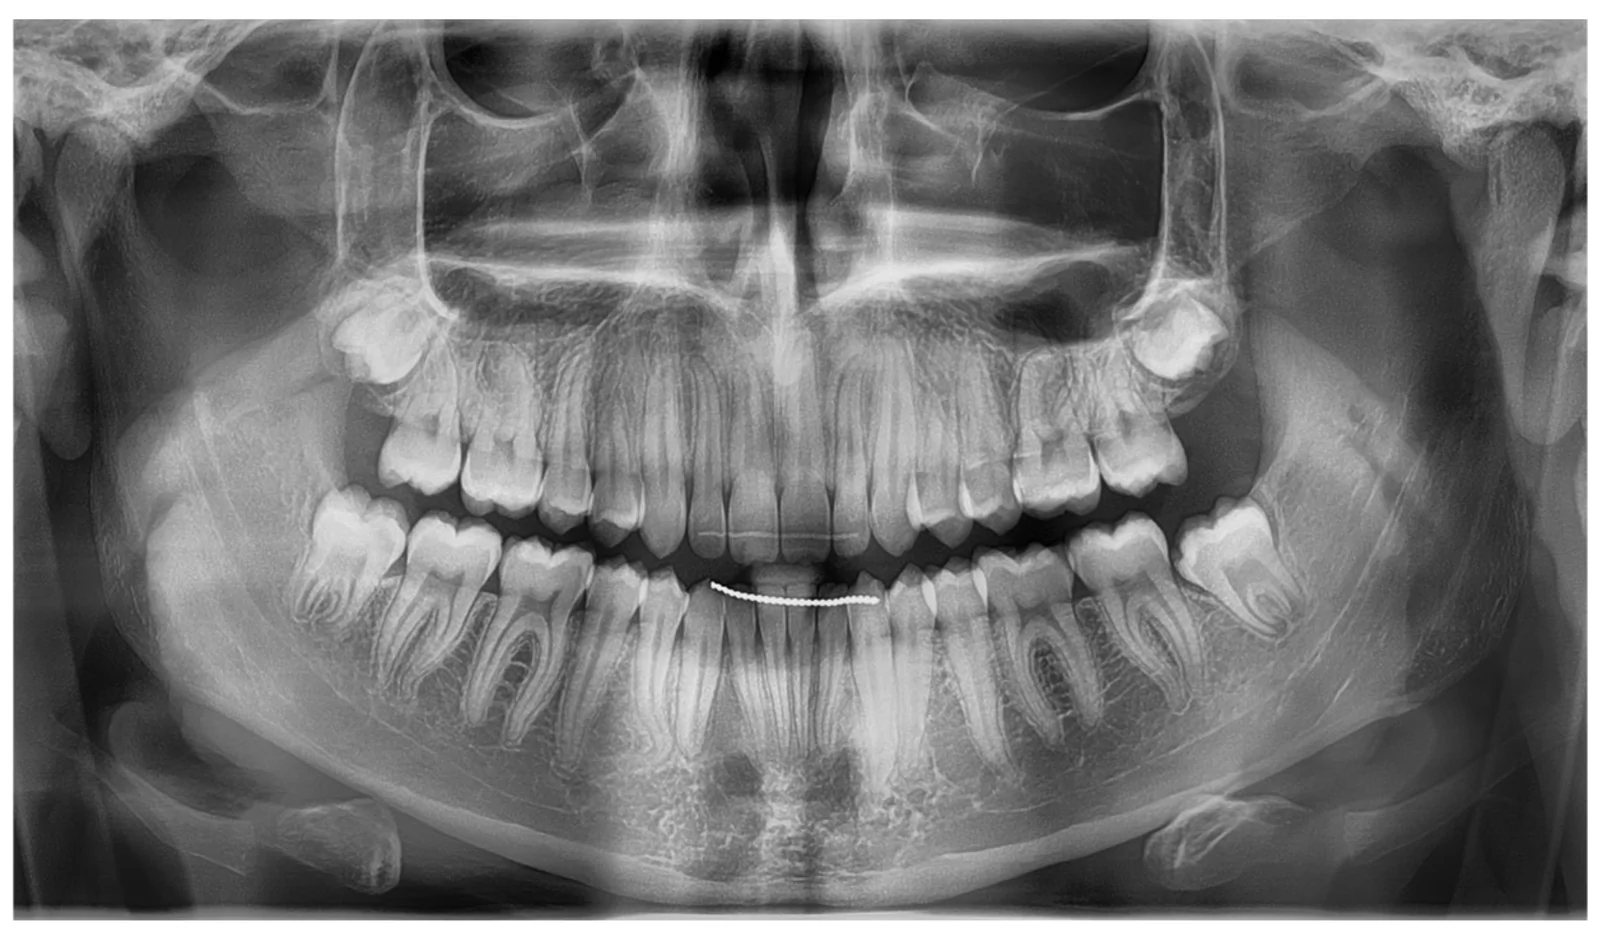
Final panoramic radiograph.
